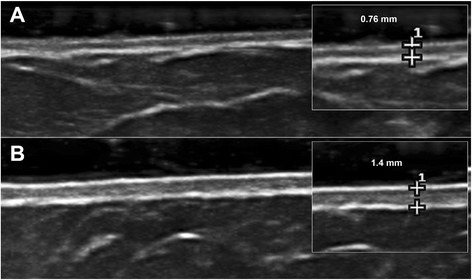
Example of measurement of dermal thickness by skin high-frequency ultrasound (18 MHz probe) in a healthy subject (a) and in a patient with systemic sclerosis (b) at the level of the abdomen
